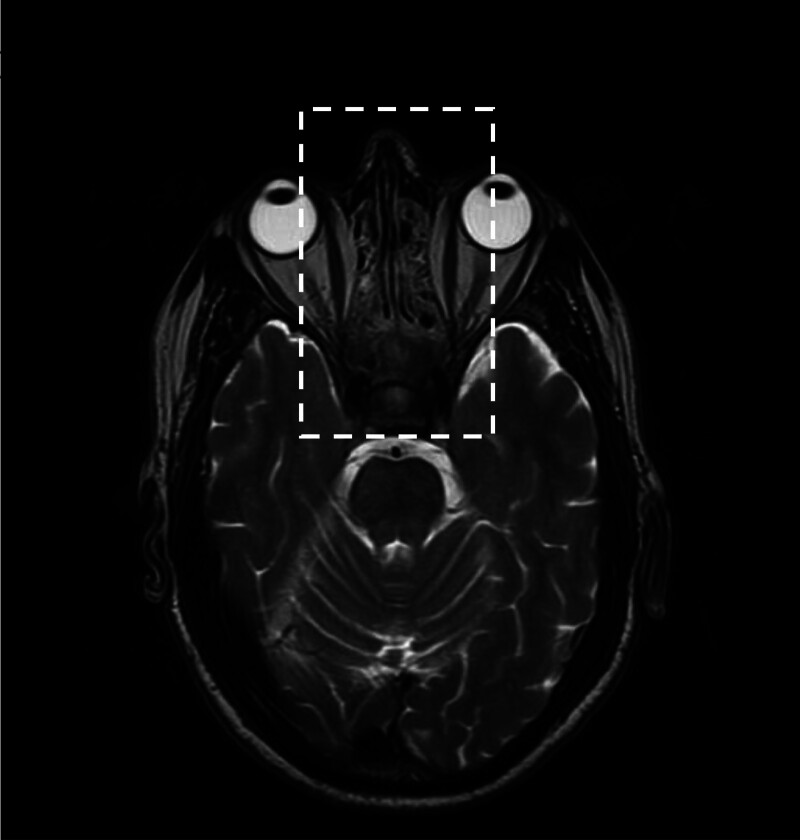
Brain MRI showing diffuse remodeling of the clivus and the ethmoid bone. The figure is captured by a brain MRI in T2 weighted-sequence. The dashed box frames diffuse remodeling of the clivus and the ethmoid bone. MRI = magnetic resonance imaging.
